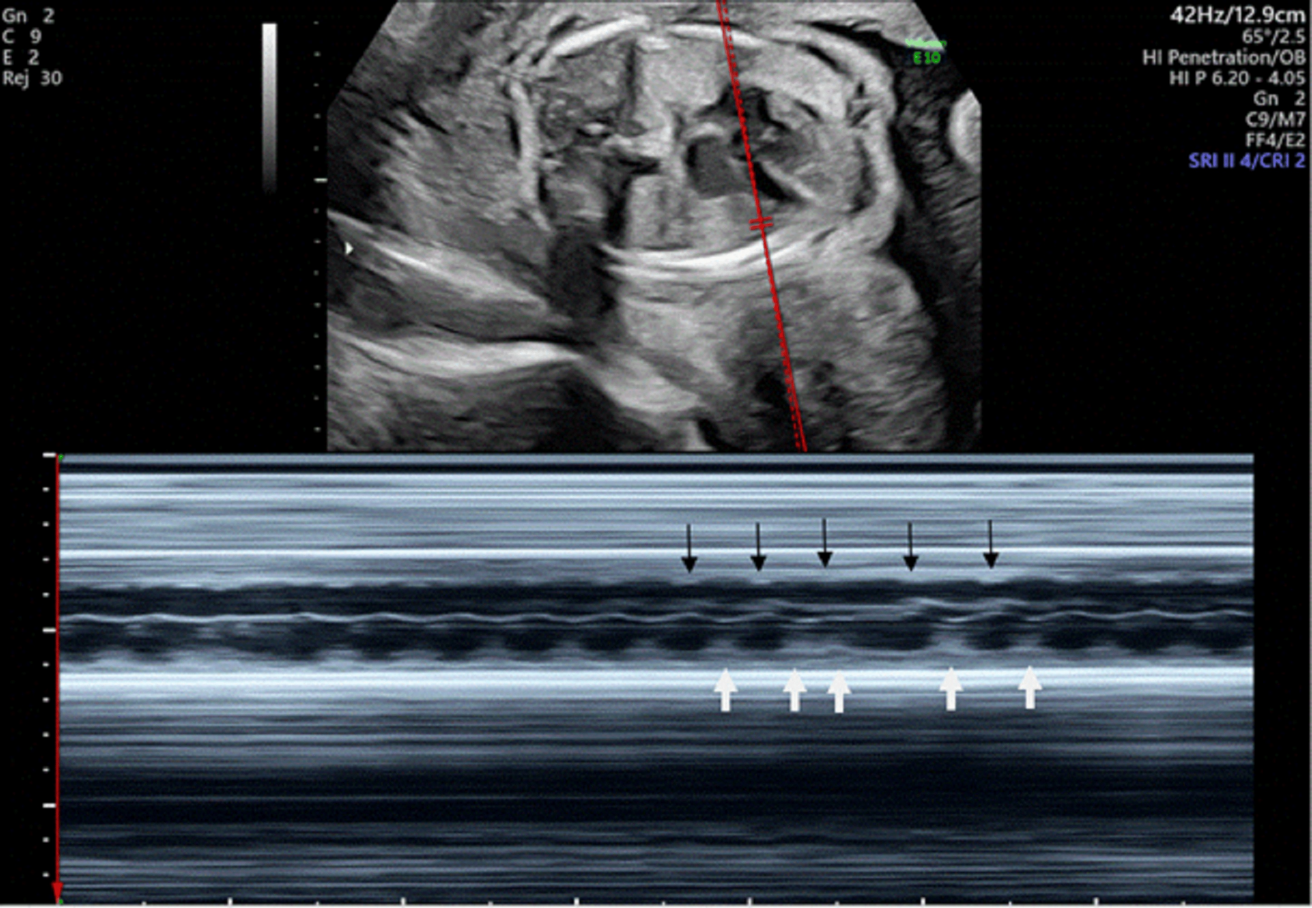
M-mode echocardiographic tracing of the fetal atrium and ventricle demonstrating a premature ventricular contraction Atrial contraction occurs independently of the ectopic ventricular beat. The black arrow denotes an atrial event, and the white arrow denotes a ventricular event. A compensatory pause follows the premature ventricular contraction.
